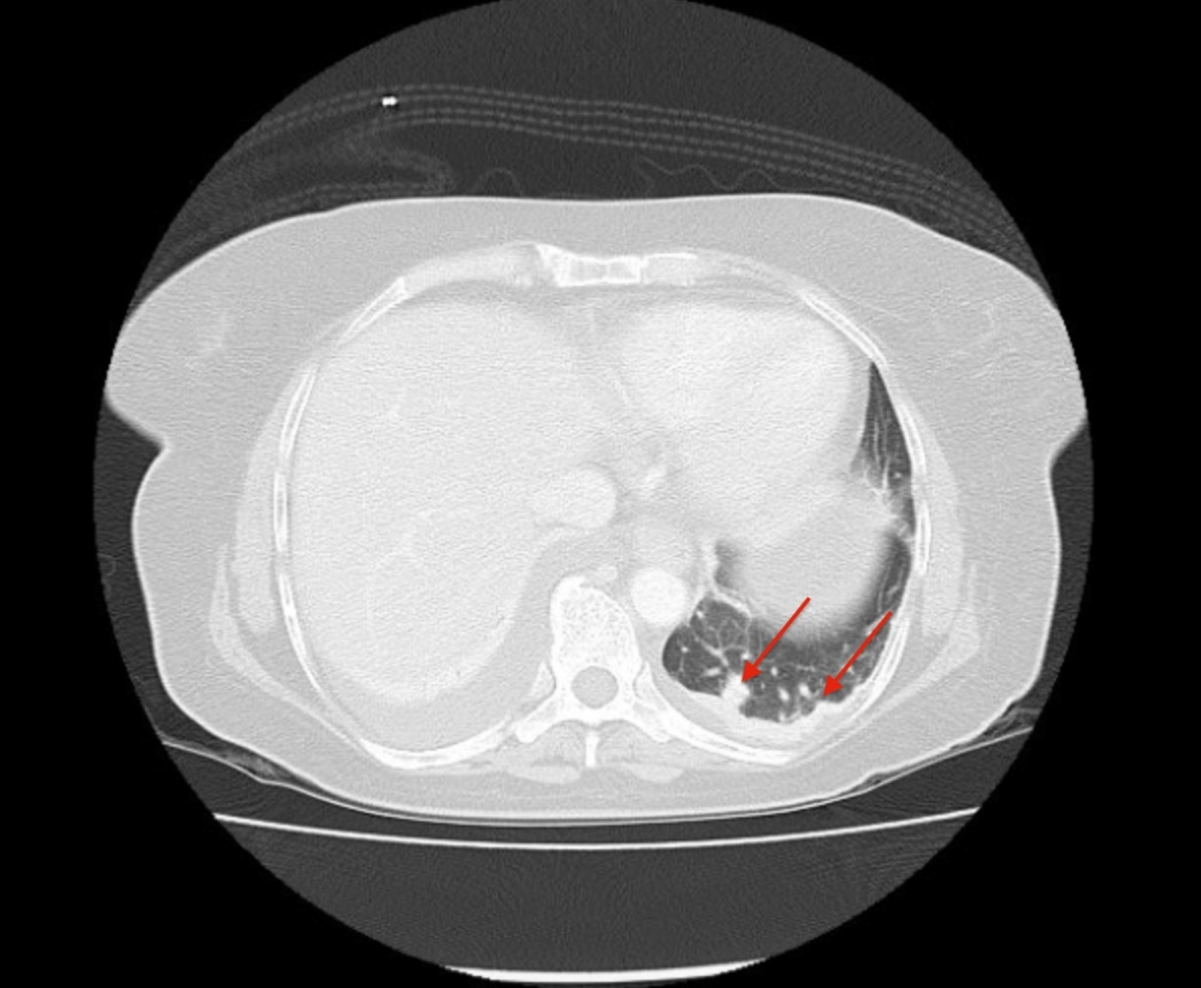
Axial section of the patient's CT scan showing nodules in the lower left lung (red arrows) CT: computed tomography
